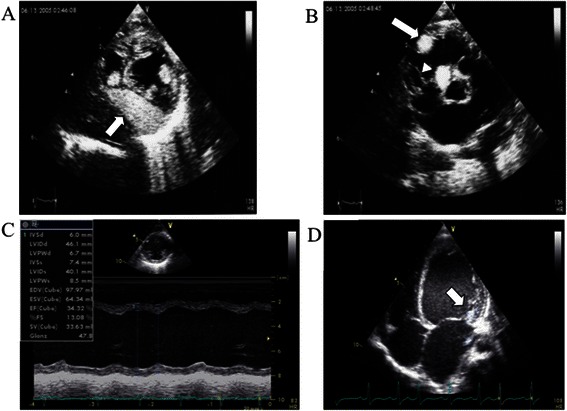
Echocardiographic findings. At birth, tumors were located on the postero-septal wall of the left ventricle (a, *arrow*), right ventricular outflow (b, *arrow*), and intraventricular septum (b, *arrowhead*) at birth. At the age of 23 months, 2D echocardiography disclosed an enlarged left ventricle (c), and M-mode echocardiography revealed poor left ventricular wall contraction (d)
